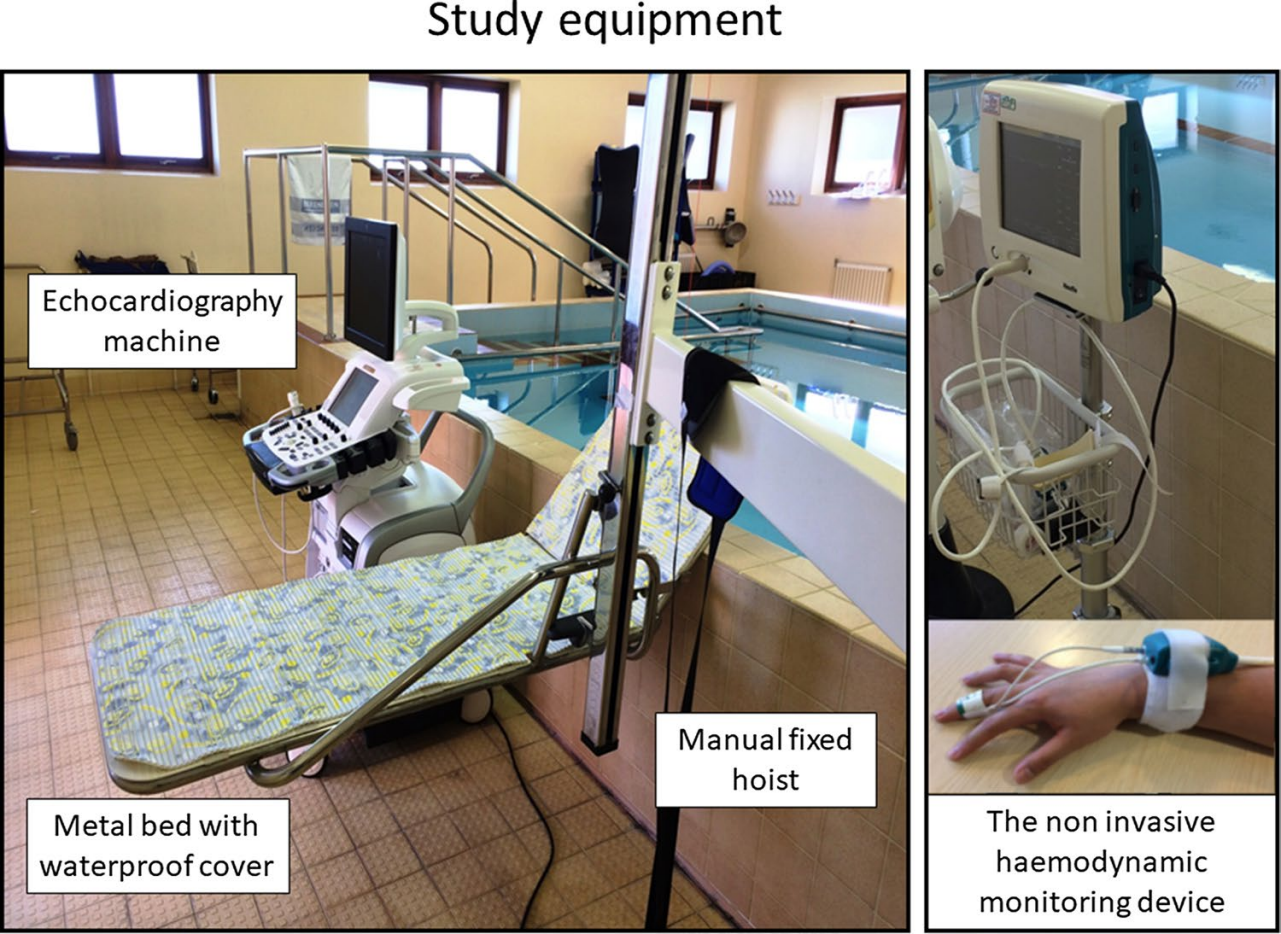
Study equipment. Echocardiography machine: GE Vivid E9, Hatfield, Hertfordshire, UK. Non-invasive haemodynamic monitoring device (Nexfin, BMeye, Amsterdam, The Netherlands) with an inflatable finger cuff was attached to the mid finger of participant’s left hand. Hydrotherapy pool (maintained at a temperature between 33 and 35 °C)
